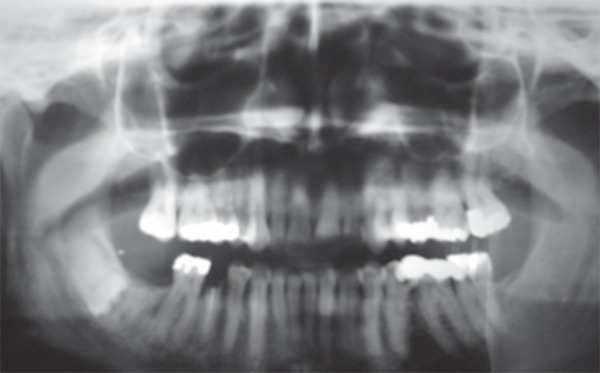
Panoramic radiograph showing a radiopaque lesion in the right oral mucosa
